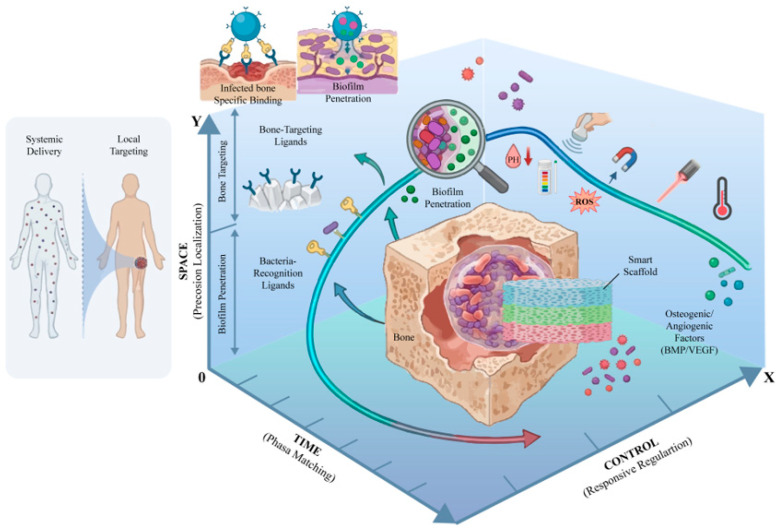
A “Time–Space–Control” coordinate system for programmable therapy in infected bone defects. Time (phase matching): a phase-aligned release profile with an early antibiofilm/antibacterial burst is followed by sustained or delayed pro-angiogenic/osteogenic delivery to avoid temporal mismatch. Space (precision localization): bone- and bacteria-targeting ligands enable defect retention, interface enrichment, and biofilm penetration, achieving high local exposure with reduced systemic spillover. Control (responsive regulation): pathology-conditional internal cues (low pH, ROS, enzymes) trigger on-site release, while clinician-applied external triggers (ultrasound, magnetic fields, light, temperature) provide on-demand, repeatable modulation for adaptable programmable release from a smart scaffold/carrier. Created in BioRender. Jinqiu Tian. (2026) https://BioRender.com/qekmwwl (accessed on 6 March 2026).
